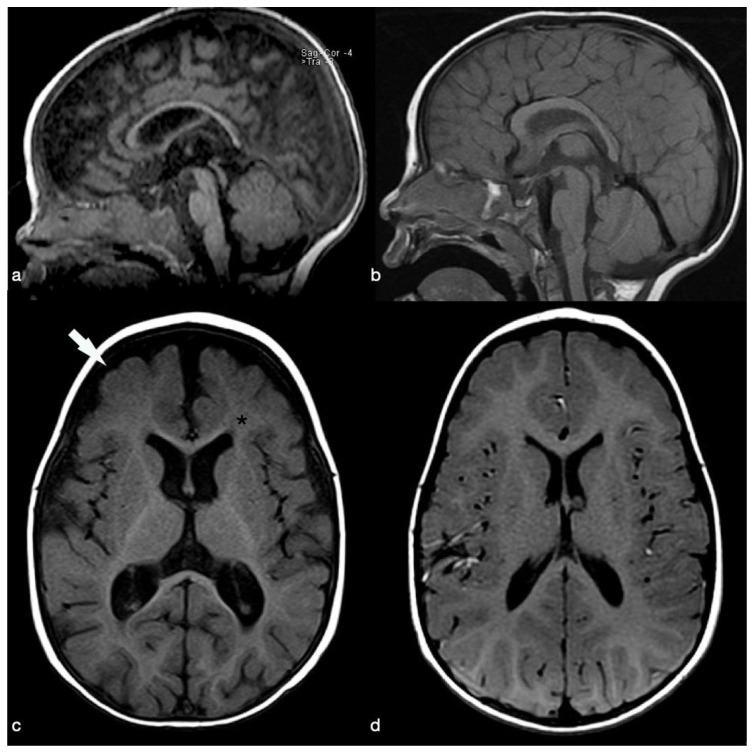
(a,b) Sagittal and (c,d) axial T1-weighted images of (a–c) the admission and follow-up MRI exams. Enlarged subarachnoid spaces (arrow in (c)) and lateral ventricles. Myelination is considered normal for the patient’s age (asterisk in (c)). (b,d) Substantial recovery of the structural changes after therapy, (c,d) an improvement of the white matter amount is evident in particular.
